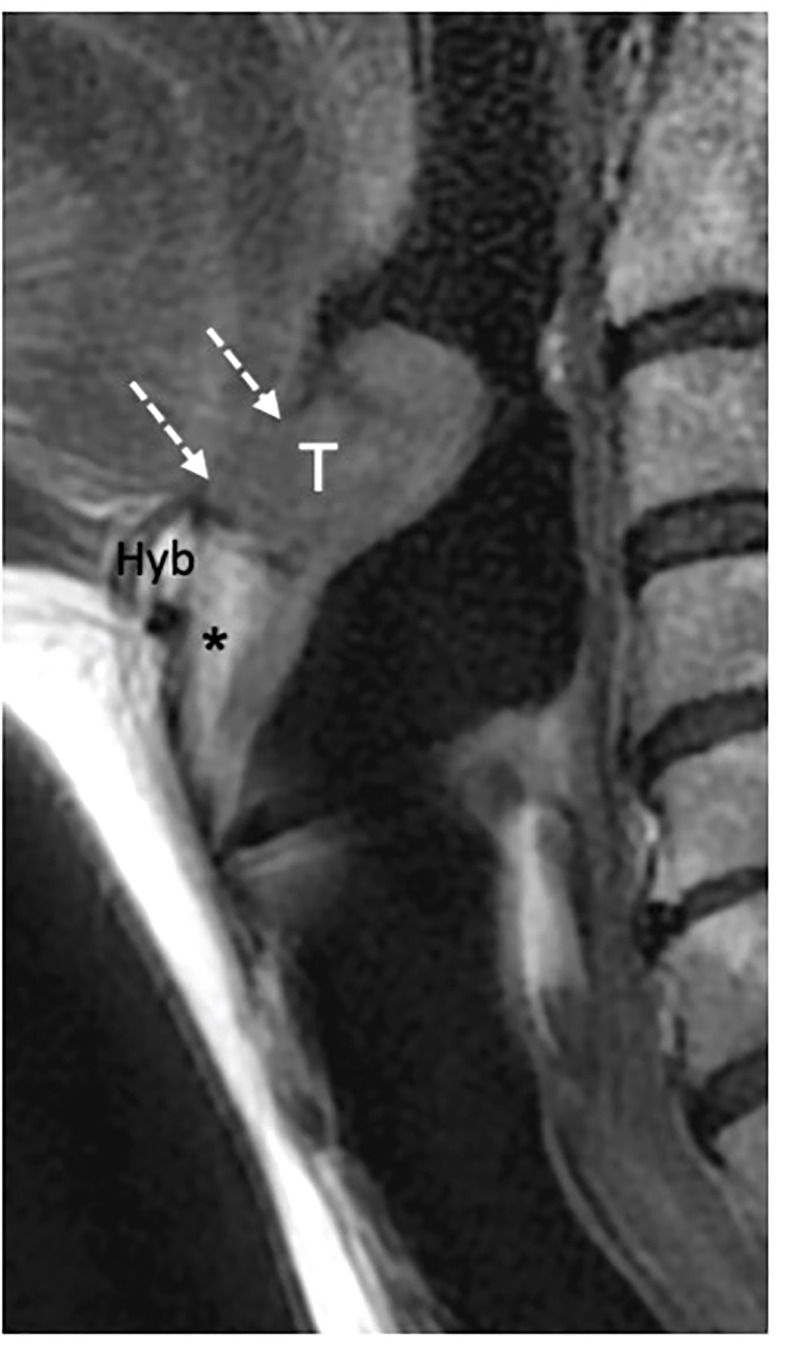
MRI T2-weighted image on sagittal piane shows a supraglottic tumor (T) involving the linguai surface of the suprahyoid epiglottis. The lesion is adjacent to the base of tongue (dashed white arrows) that is not involved. Hyoid bone (Hyb) is normal as well as the pre- epiglottic space (asterisk).
